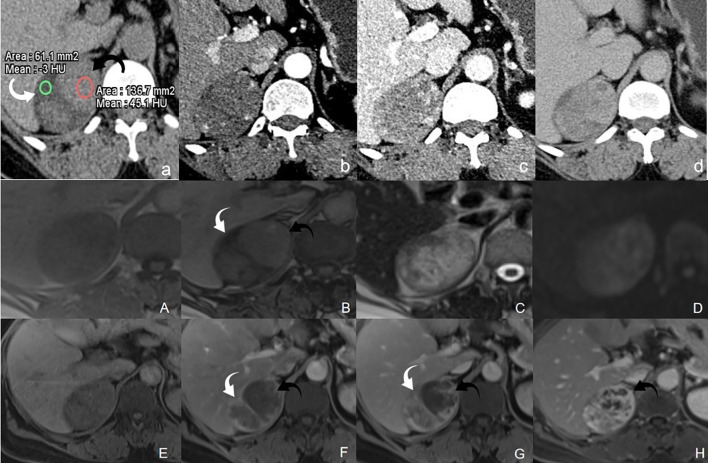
CT scan shows a well-defined, oval mass in the right adrenal gland. (A) The mass showed heterogeneous density: the hyperdense area had a CT value of 36~44.4 HU (black arrows), while the hypodense area measured -4.6~-2.4 HU (white arrows). (B-D) On enhanced imaging, the hyperdense area exhibited persistent progressive enhancement, whereas the hypodense area showed mild arterial-phase enhancement, increased venous-phase enhancement, and delayed-phase washout.
